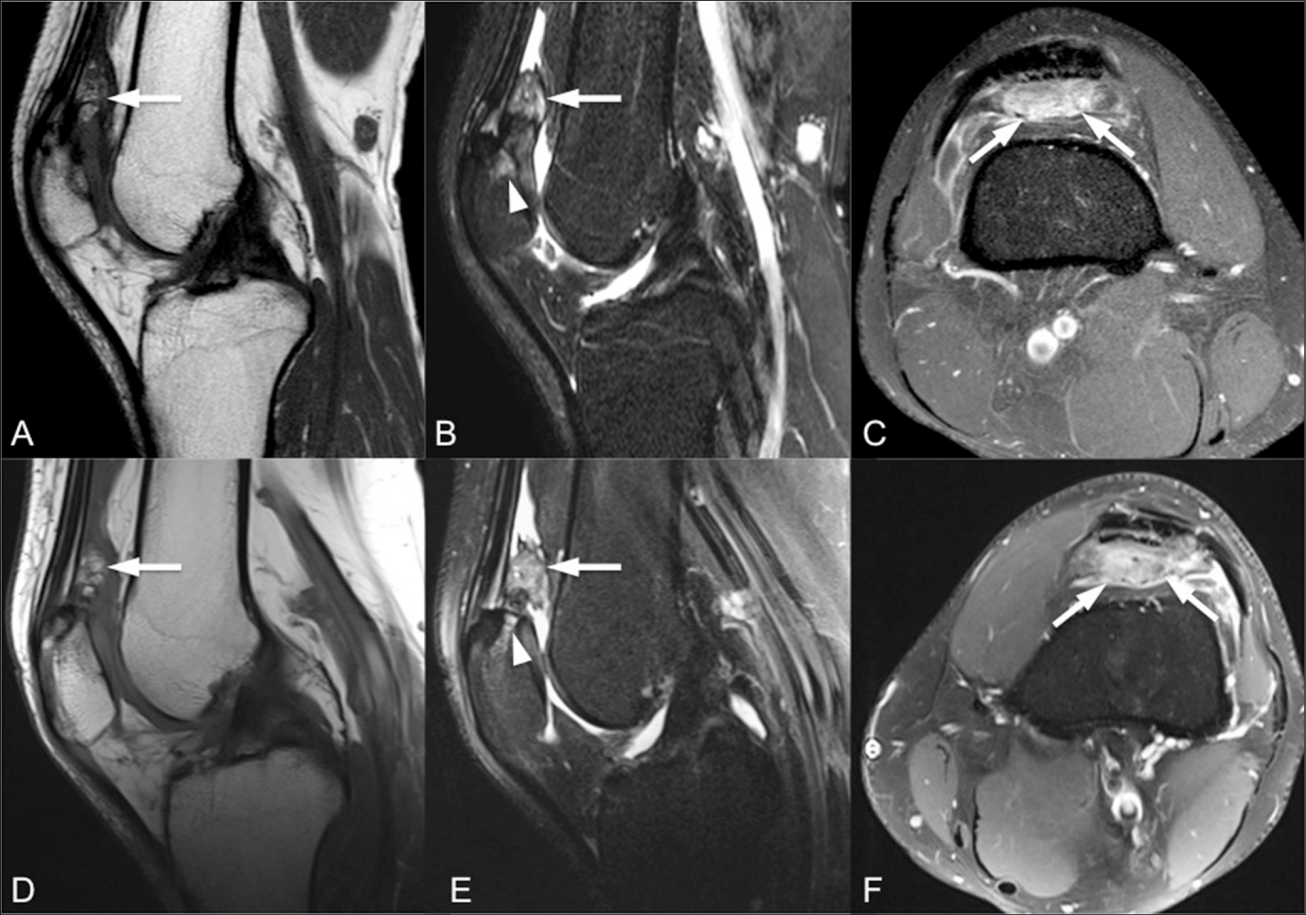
Sagittal T1-weighted (A, D), sagittal fat-saturated T2-weighted (B, E) and intravenous contrast-enhanced axial fat-saturated T1-weighted (C, F) MR images (top row for right knee, bottom row for left knee) show enlarged and inflamed quadriceps fat pad indicated by increased signal intensity with convex posterior border and contrast enhancement by intravenous contrast administration (arrows).
